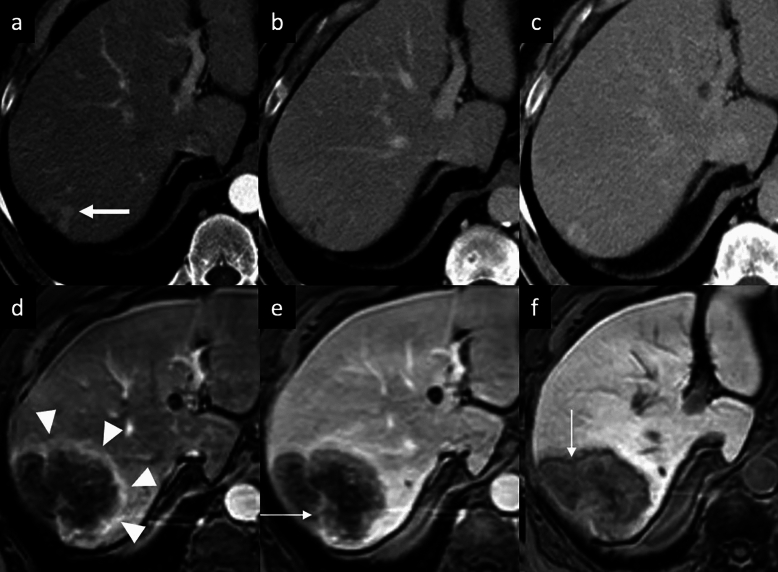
Intrahepatic mass-forming cholangiocarcinoma in a 74-year-old man with past medical history of prostatectomy who presented mild elevation of liver enzymes. a–c Contrast-enhanced CT exam shows a small subcapsular focal liver lesion on the right lobe, displaying a peripheral enhancement on arterial phase (arrow, a), that progresses on b portal venous and c delayed phases. On gadoxetic acid-enhanced MRI follow-up at 3 years the lesion increases in size and it shows a peripheral rim enhancement on arterial phase (arrowheads, d), with central intralesional enhancement on portal venous phase (thin arrow, e). f On hepatobiliary phase the tumor displays a “targetoid appearance”, with a central cloud-like hyperintensity surrounded with a low signal intensity peripheral rim (arrow, f)
